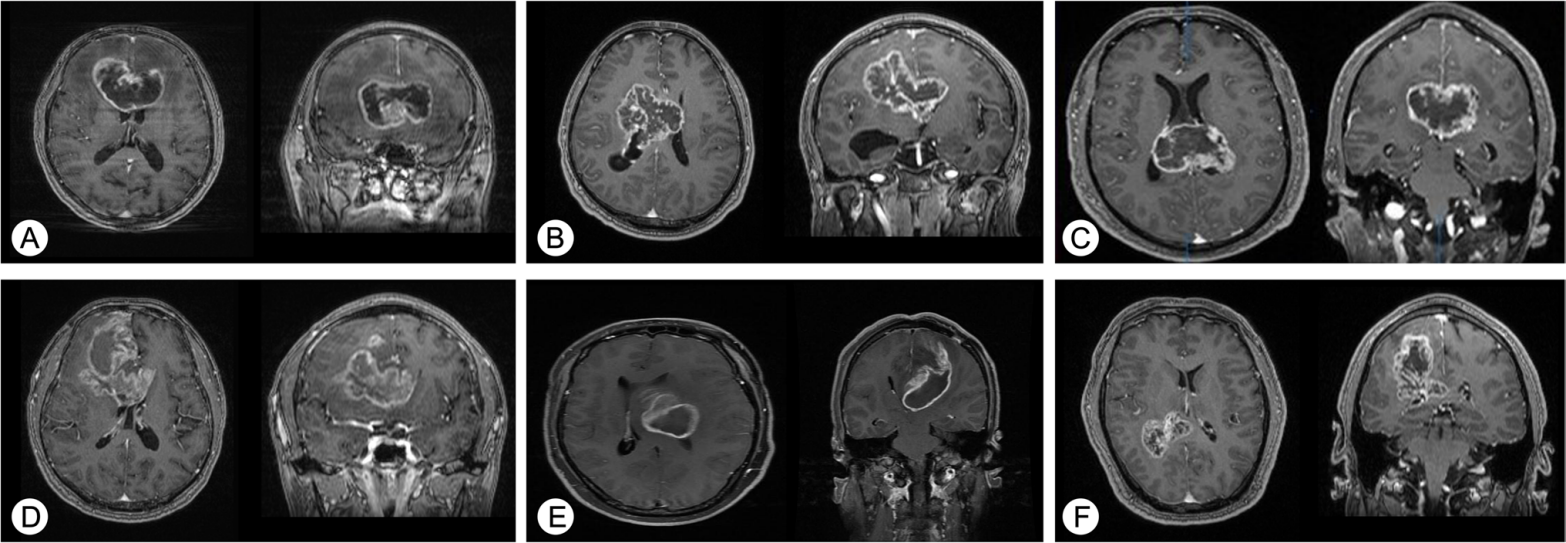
Characteristics of ccGBM that invaded different parts of the corpus callosum. All images originated from axial and coronal postcontrast 3D T1-weighted MRI. Genu invasion of the CC (A, D), body invasion of the CC (B, E), and splenium invasion of the CC (C, F). The upper column shows the bGBM (A–C); the lower column shows the non-butterfly ccGBM (D–F)
